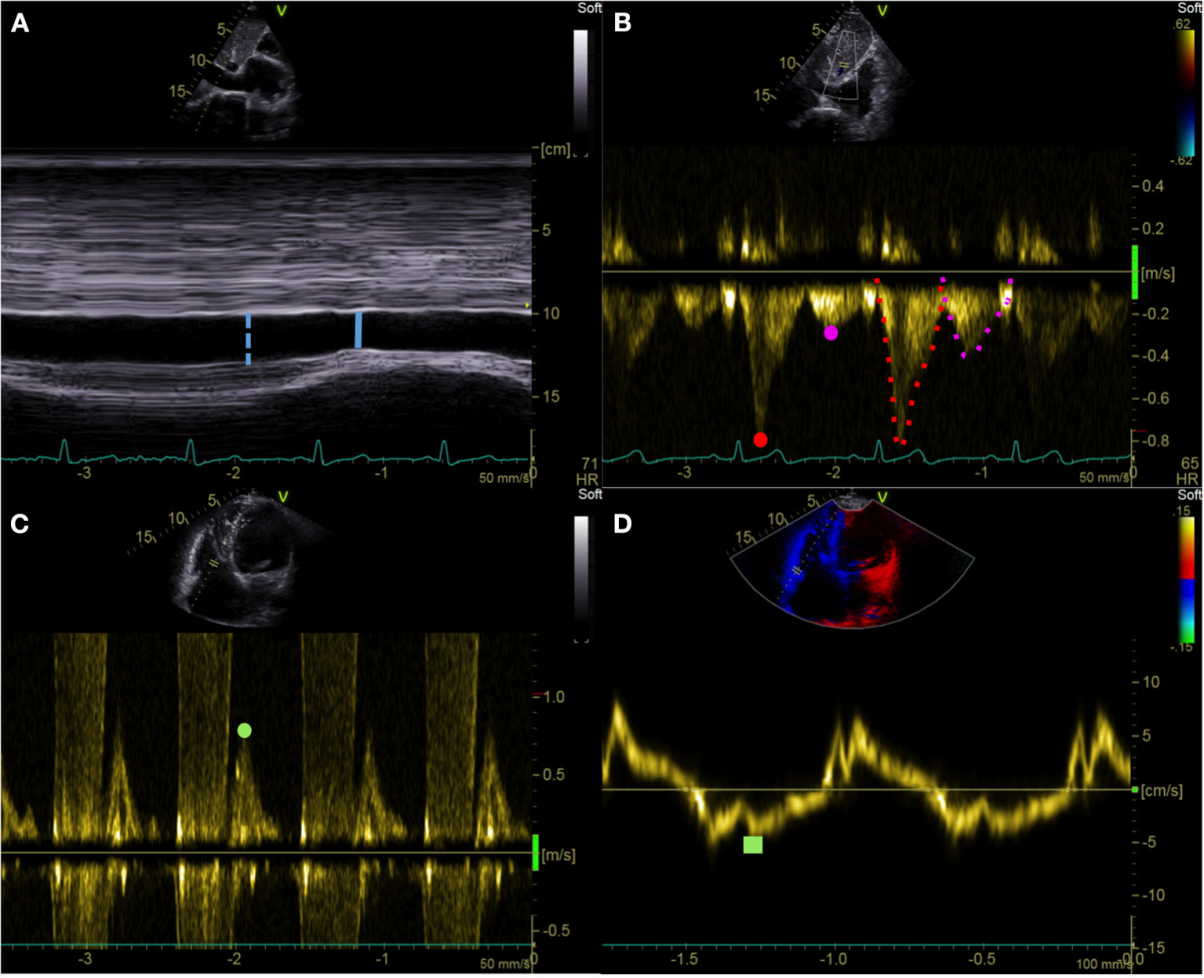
Echocardiographic right atrial pressure estimation. (A) IVC end-expiratory diameter (blue dotted line) and respiratory variation (solid line). (B) HV pulsed wave Doppler assessment: Vs (red dot), Vd (purple dot), VTIs (red dotted line), and VTId (purple dotted line). (C,D) Tricuspid *E*/*e*′ ratio: pulsed wave Doppler tricuspid inflow early E-wave peak velocity (green dot) and tricuspid lateral annulus tissue Doppler imaging *e*′ wave velocity (green square). IVC, inferior vena cava; HV, hepatic vein; Vs, hepatic vein peak systolic velocity; Vd, hepatic vein peak diastolic velocity; VTIs, velocity-time interval of the HV systolic wave; VTId, velocity-time interval of the HV diastolic wave.
